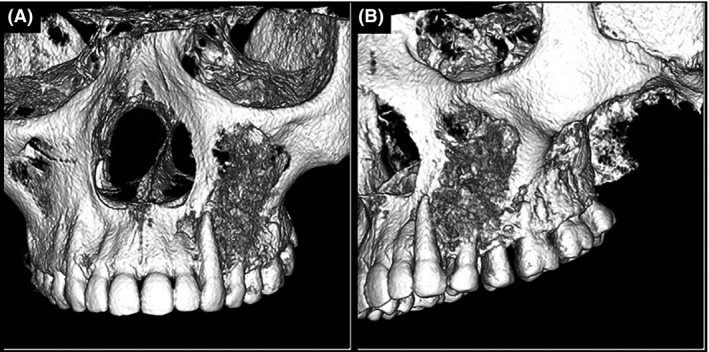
(A and B) CBCT 3D reconstruction of the maxilla showing buccal cortical plate destruction with numerous spicules of calcifications on the left side.
